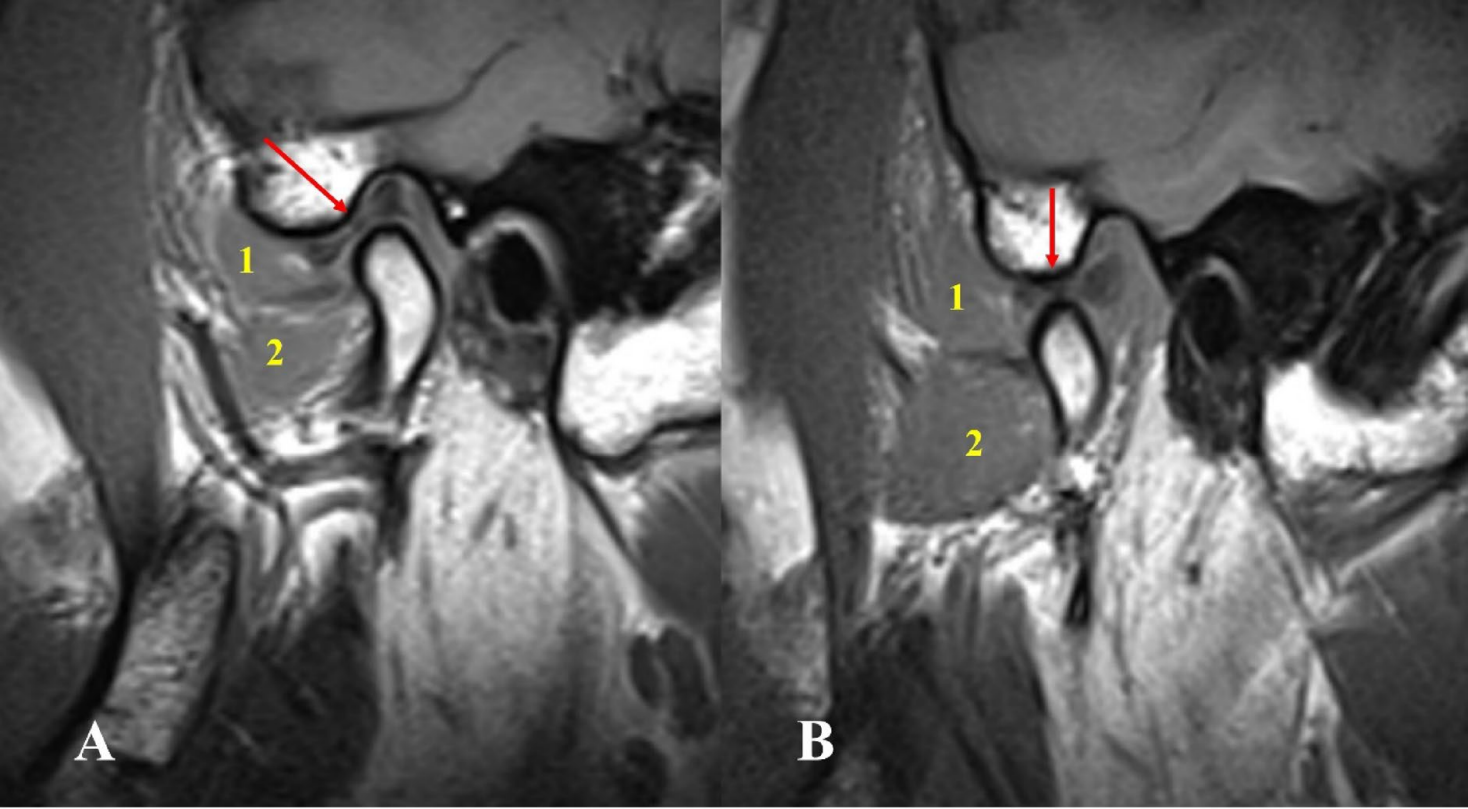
Oblique sagittal T1-weighted images represent Type II-B with two heads of lateral pterygoid muscle, with upper attached to the disc and condyle and the lower to the condyle.
